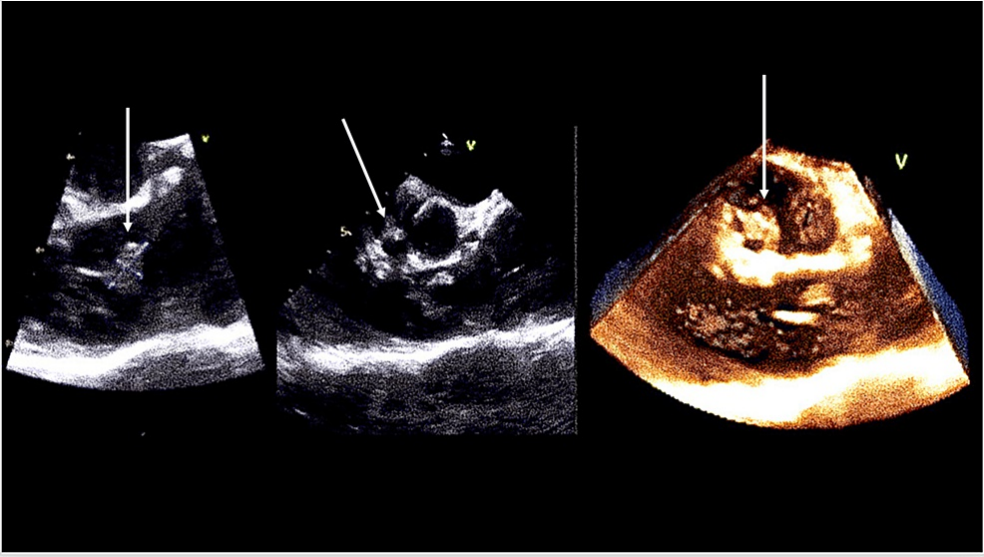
TEE images showing vegetation in the PV. TEE, transesophageal echocardiography; PV, pulmonic valve
